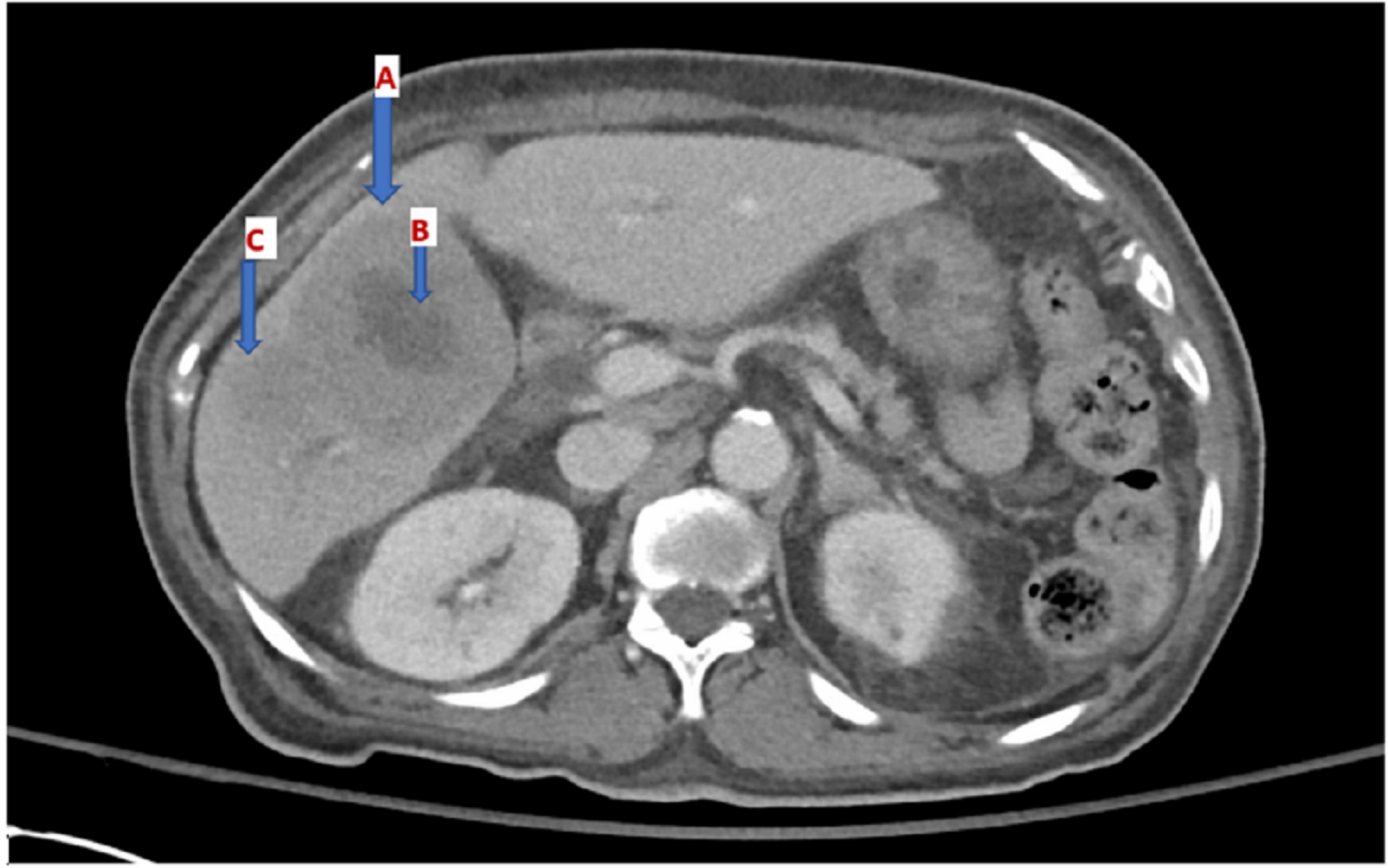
CT of the abdomen and pelvis depicting two hypoenhancing masses. Arrow A shows 8.8 × 7.1 × 5.7 cm hypoenhancing lesion in the right lobe of liver. Arrow B shows area of central necrosis. Arrow C shows 6.0 × 5.8 × 5.3 cm lesion.
